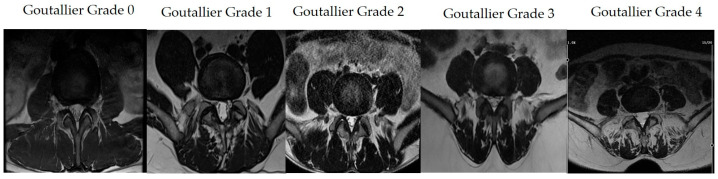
Goutallier classification on MRI images.
